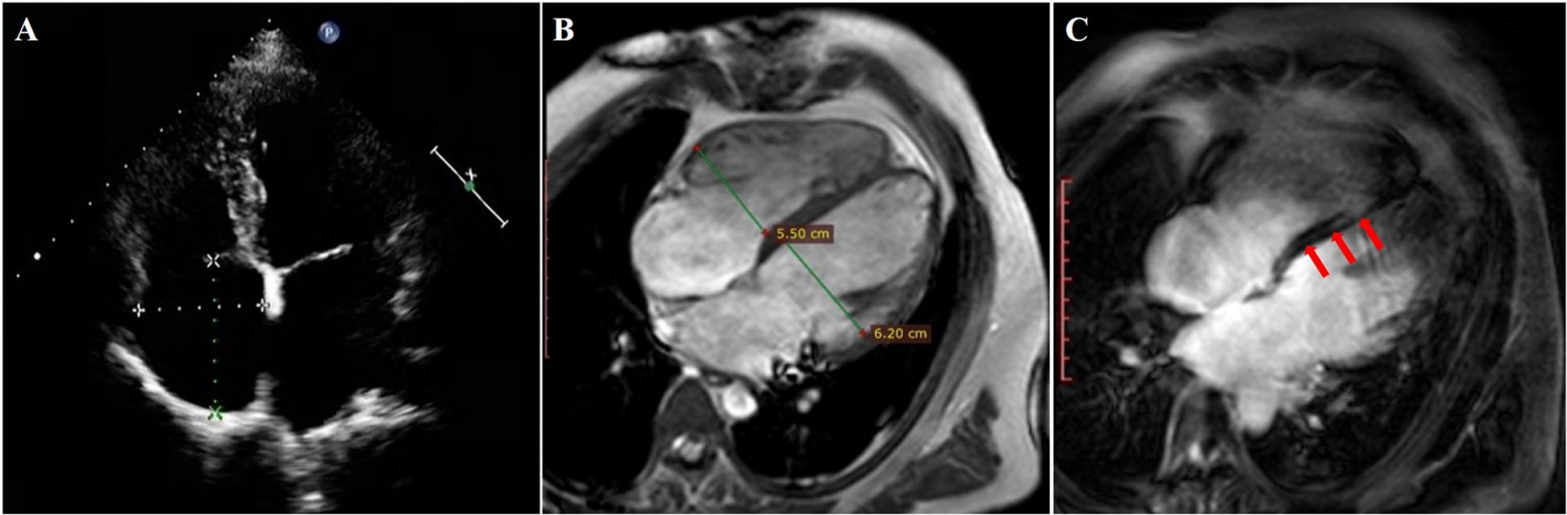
a. Transthoracic echocardiography (TTE) showing cardiac dilatation. The left atrial diameter was 44 mm, the left ventricular end diastolic diameter was 60 mm, and the left ventricular ejection fraction was 58%. b. Cardiac magnetic resonance imaging showing that the left ventricular end diastolic diameter was 62 mm and the right ventricular end diastolic diameter was 55 mm. c. Late midmyocardium gadolinium enhancement (red arrow)
